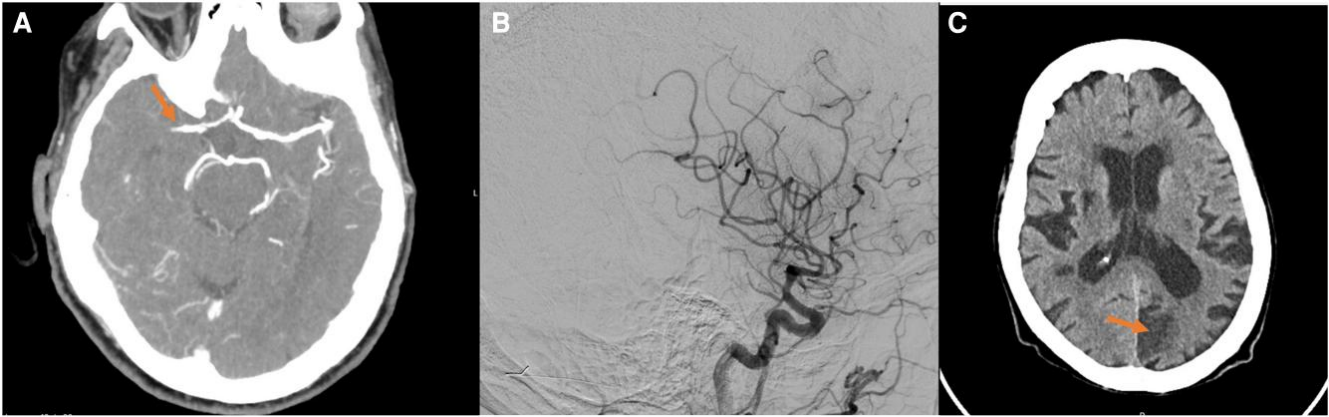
(*A*) Cerebral computed tomography showing presence of a thrombus in the proximal right M1 segment. (*B*) Cerebral angiography after successful thrombectomy. (*C*) Cerebral computed tomography following second stroke 3 months later, exhibiting subacute ischaemic lesion in the left occipital region.
